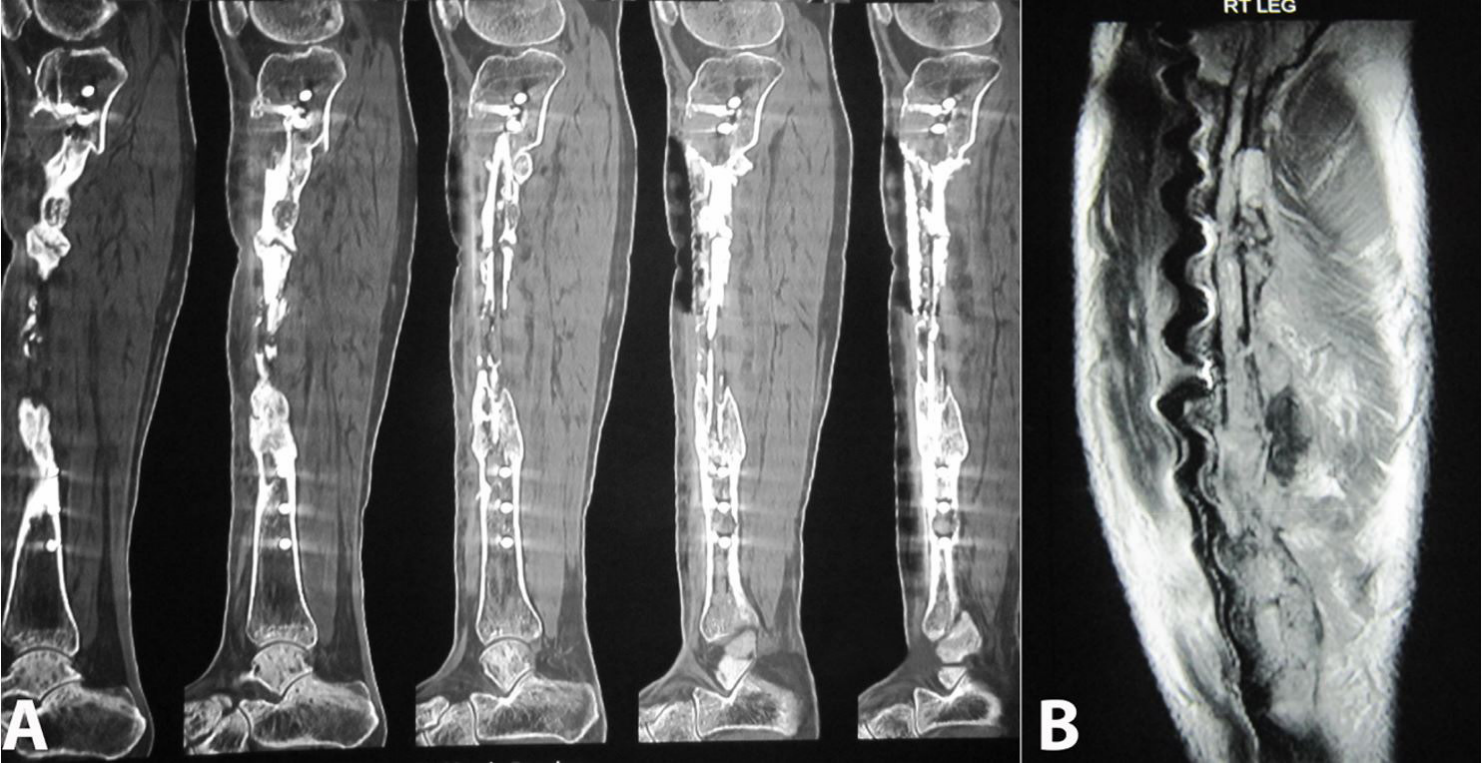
CT study of right leg: A – Sagittal sections reveal multiple patchy cortical erosions/irregularities in the right tibia centered in the diaphysis and contiguously involving the adjacent upper metaphysis. Note adjoining soft tissue thickenings. Streak artifacts are noted due to postoperative changes (Screws); B – MRI Sagittal section of right leg reveals cortical erosions/irregular outlines of the tibia with altered signals in the adjoining bone marrow and soft tissue. Susceptibility artifacts are noted due to postoperative metallic screws.
